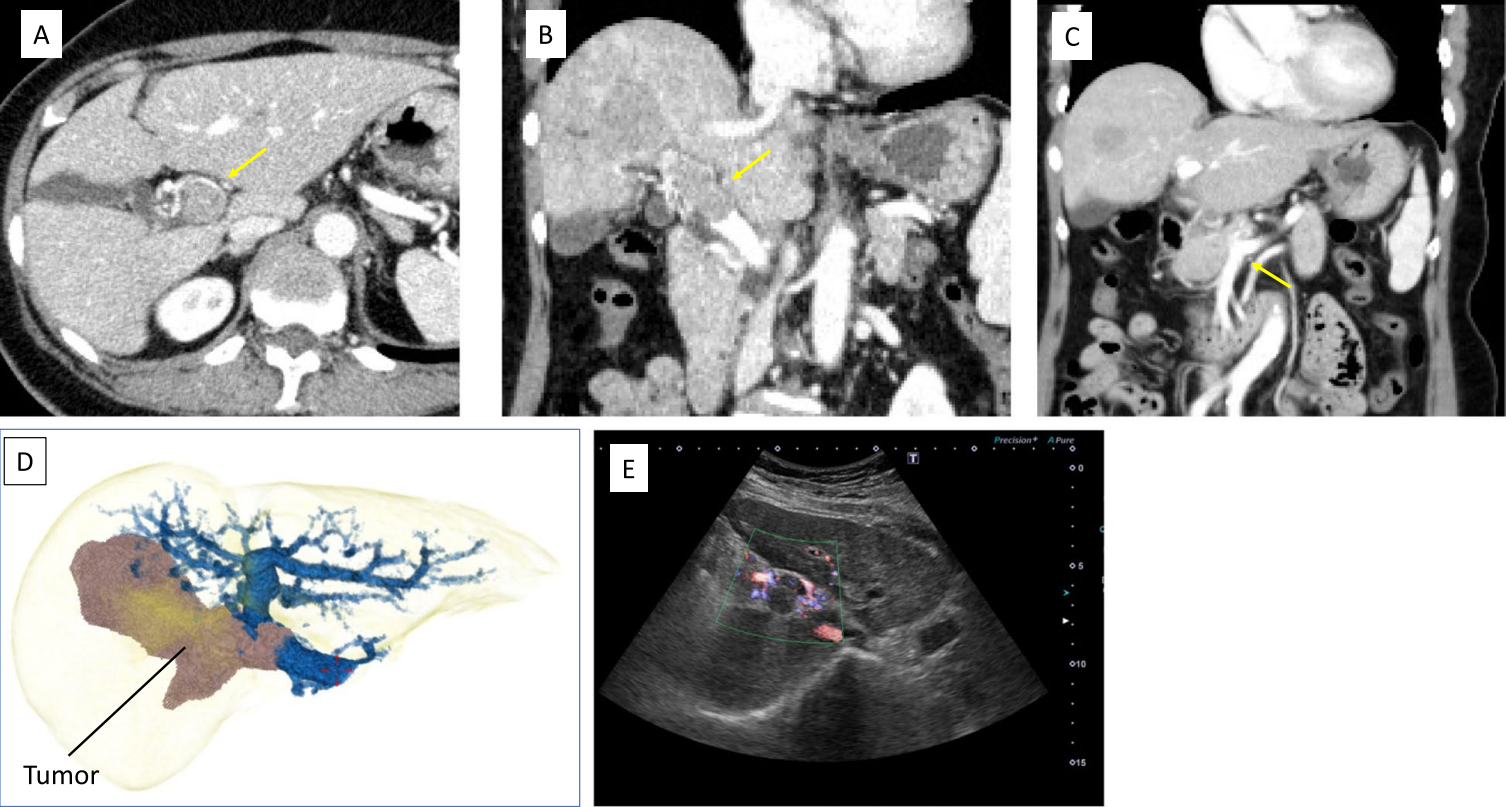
Preoperative imaging evaluating the tumor thrombus of the portal vein at the time of admission. A Axial image of the enhanced computed tomography (CT) image. B Coronal image. C Coronal image of the enhanced CT showing that the confluence of the superior mesenteric vein and splenic vein is intact. D Three-dimensional imaging showing the association between the tumor and portal vein. E Abdominal ultrasonography showing that barely any portal blood flow is present
